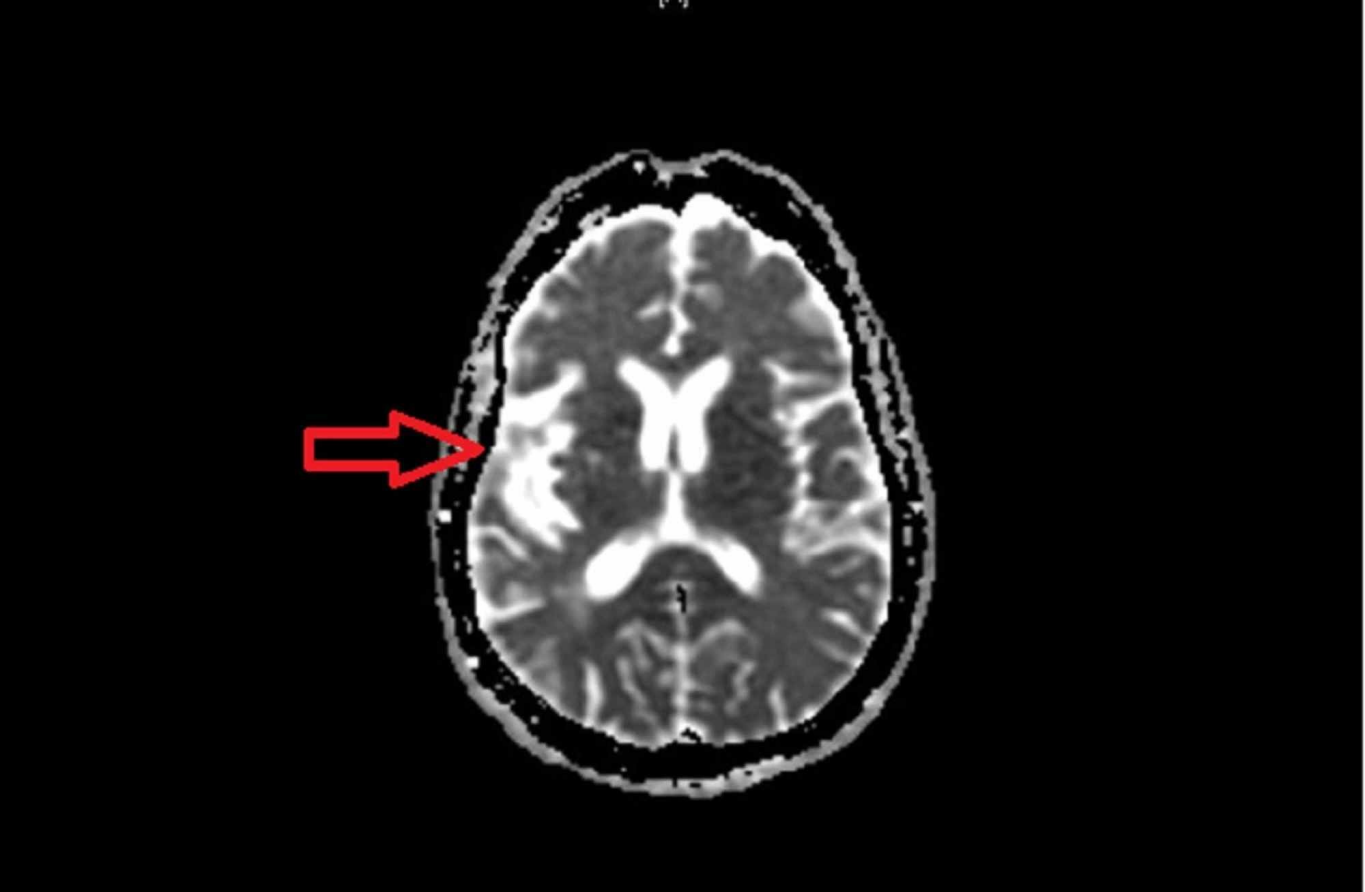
Brain MRI showed acute ischemia in the mid and post right frontal lobe
